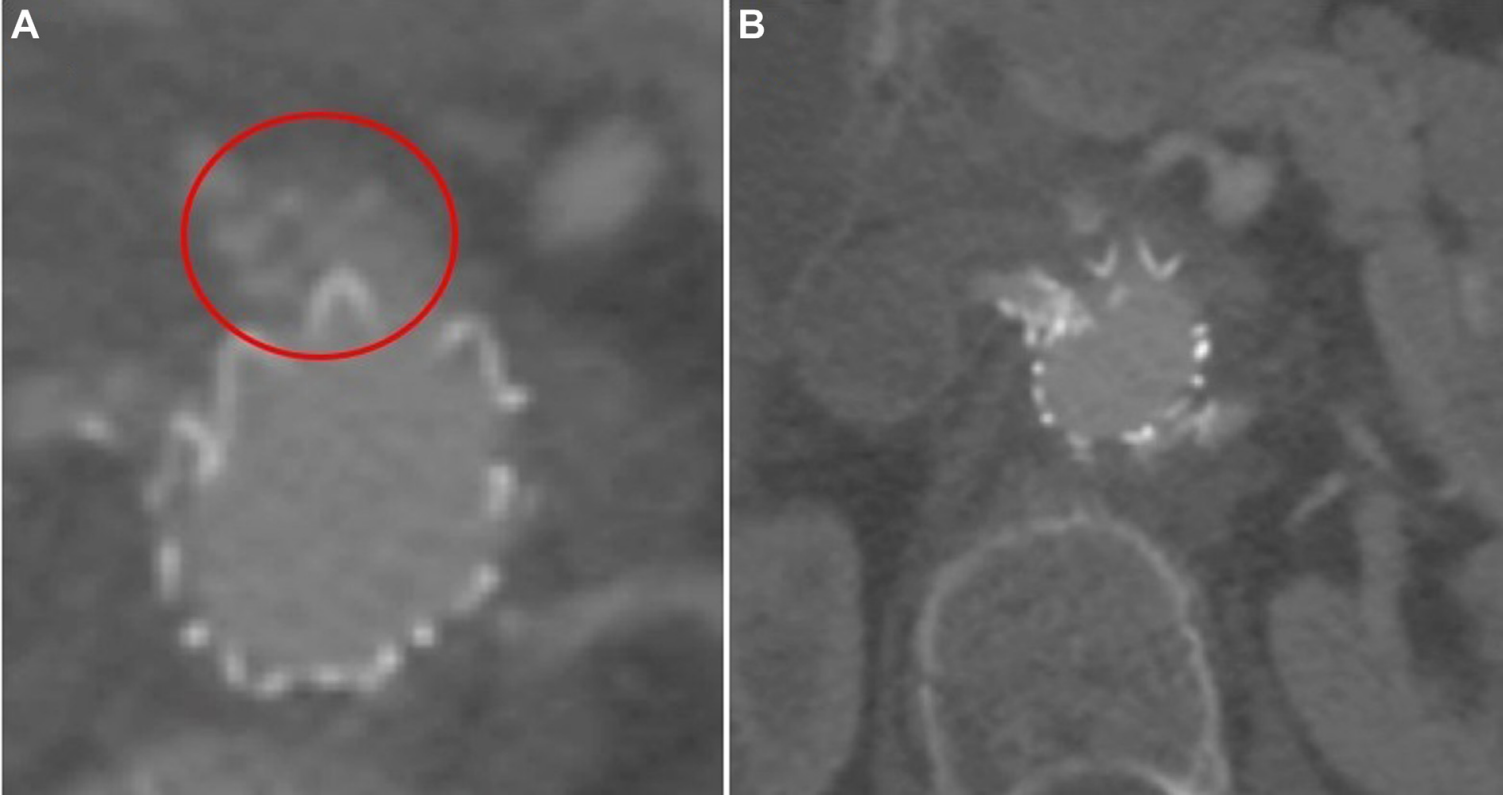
One-month follow-up computed tomography angiogram showed a persistent type IA endoleak.
